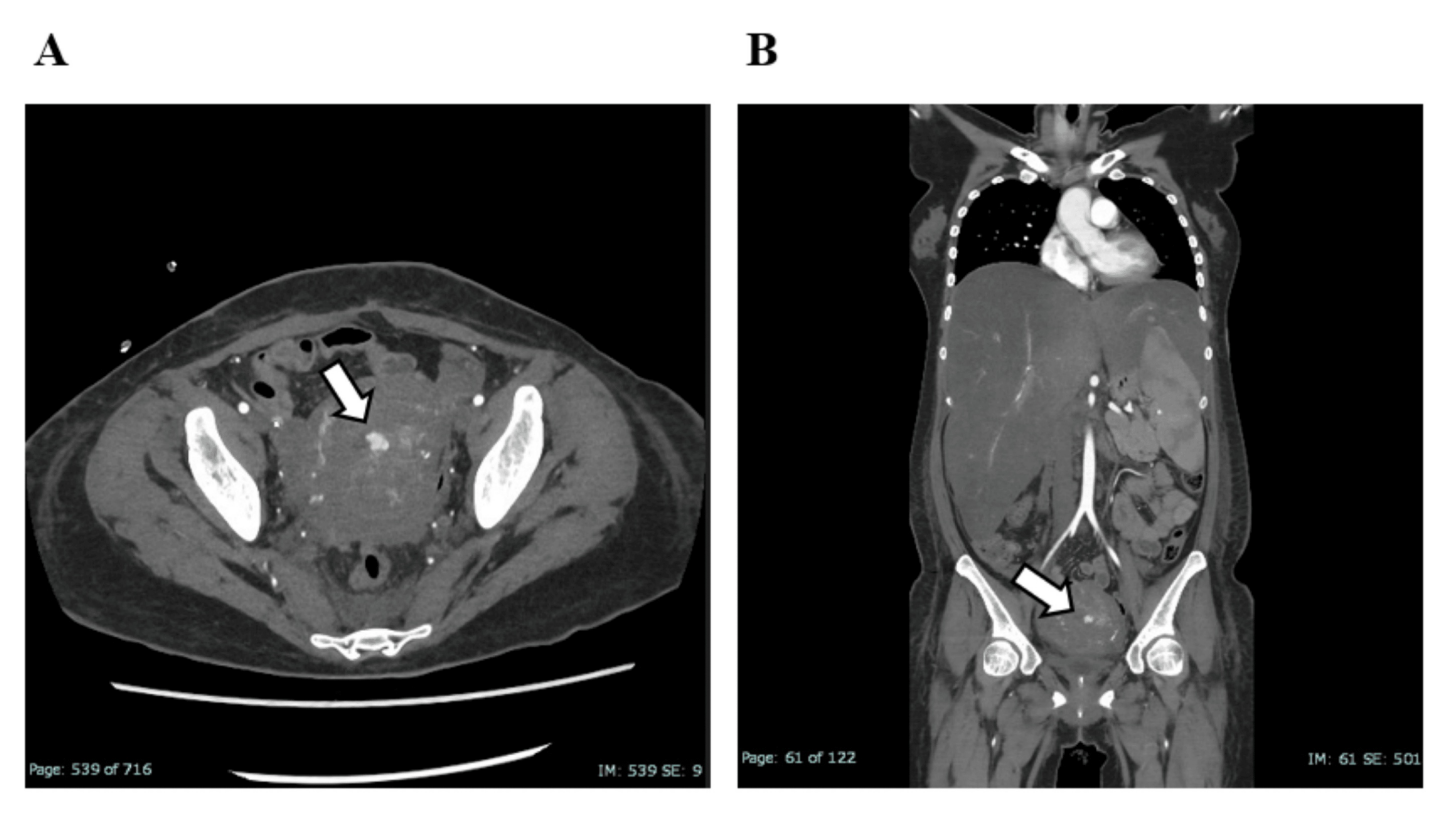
Contrast-enhanced CT showing active extravasation from the left uterine artery The images demonstrate active extravasation of contrast medium from the left uterine artery, consistent with ongoing arterial bleeding. CT: Computed tomography
